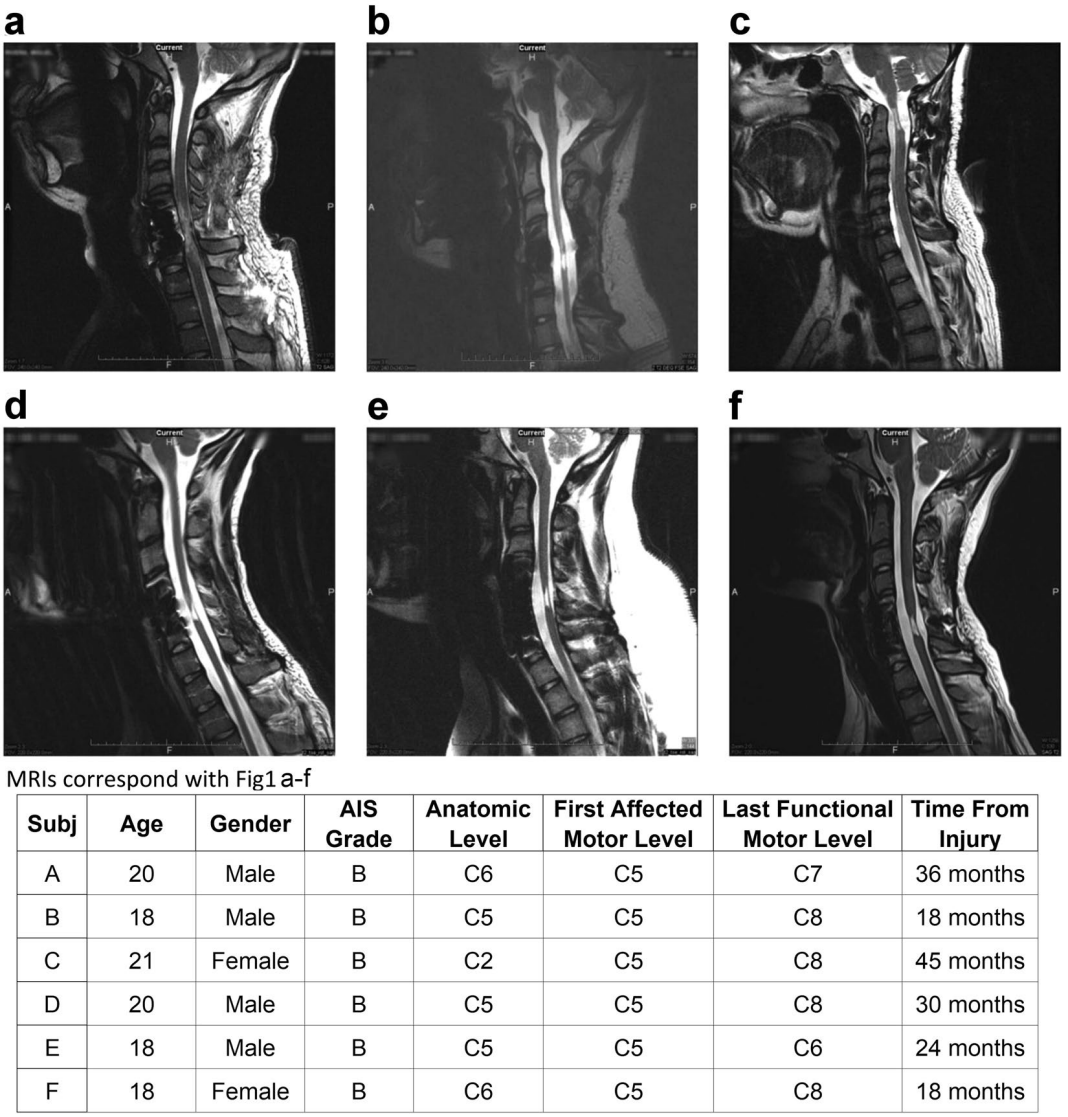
Patient demographic and functional data and sagittal T2 MRI Imaging demonstrating the location of cervical spinal cord injury of subjects (a–f) at approximately the C2 (c), C5 (b,d,e) or C6 (a,f) spinal levels. The injury location displays a high intensity T2 signal corresponding to a glial scar formation. Spinal cord tissue distal and proximal to the injury locus has a normal appearance without evidence of post-traumatic syrinx formation. The extent of cord atrophy ranges from 62% loss of cord diameter (c) to 26% loss of cord diameter (b) when compared to adjacent uninjured levels. The glial scar spans approximately one spinal cord level in all subjects with the exception of subject a where the glial scar spans two vertebral levels.
